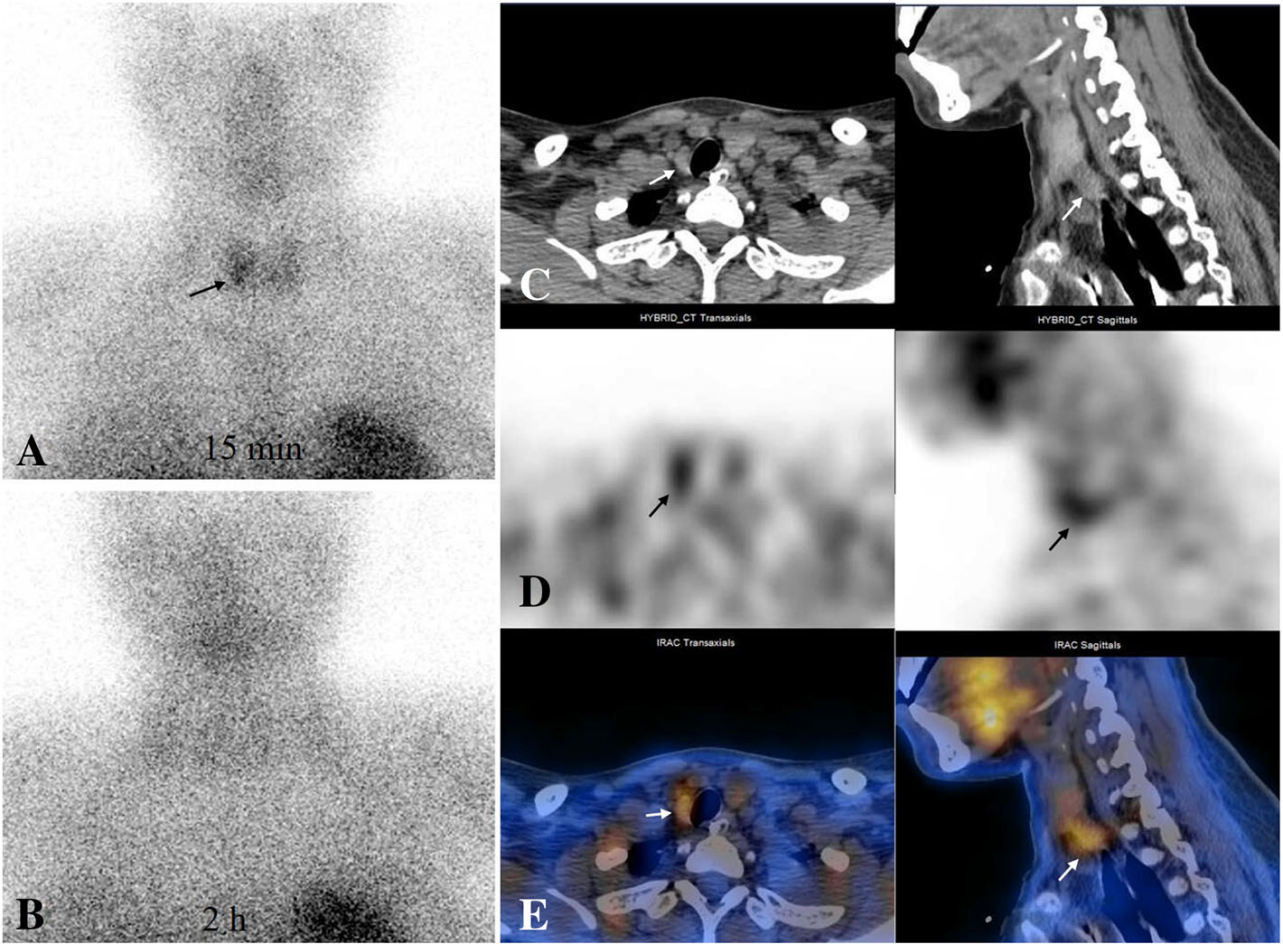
^99m^Tc-MIBI scintigraphy and SPECT/CT. ^99m^Tc-MIBI scintigraphy demonstrating increased uptake in the inferior aspect of the right thyroid lobe (arrow) on early acquisition a; rapid ^99m^Tc-MIBI clearance at 2 h delayed phase b; ^99m^Tc-MIBI SPECT/CT acquisition depicting intense radiotracer accumulation (arrow) localizing to an oval nodule, consistent with a right inferior parathyroid adenoma (c: axial and sagittal CT; d: axial and sagittal SPECT; e: fused axial and sagittal images). ^99m^Tc-MIBI = technetium-99 m-sestamibi; SPECT/CT = single photon emission tomography/computed tomography
